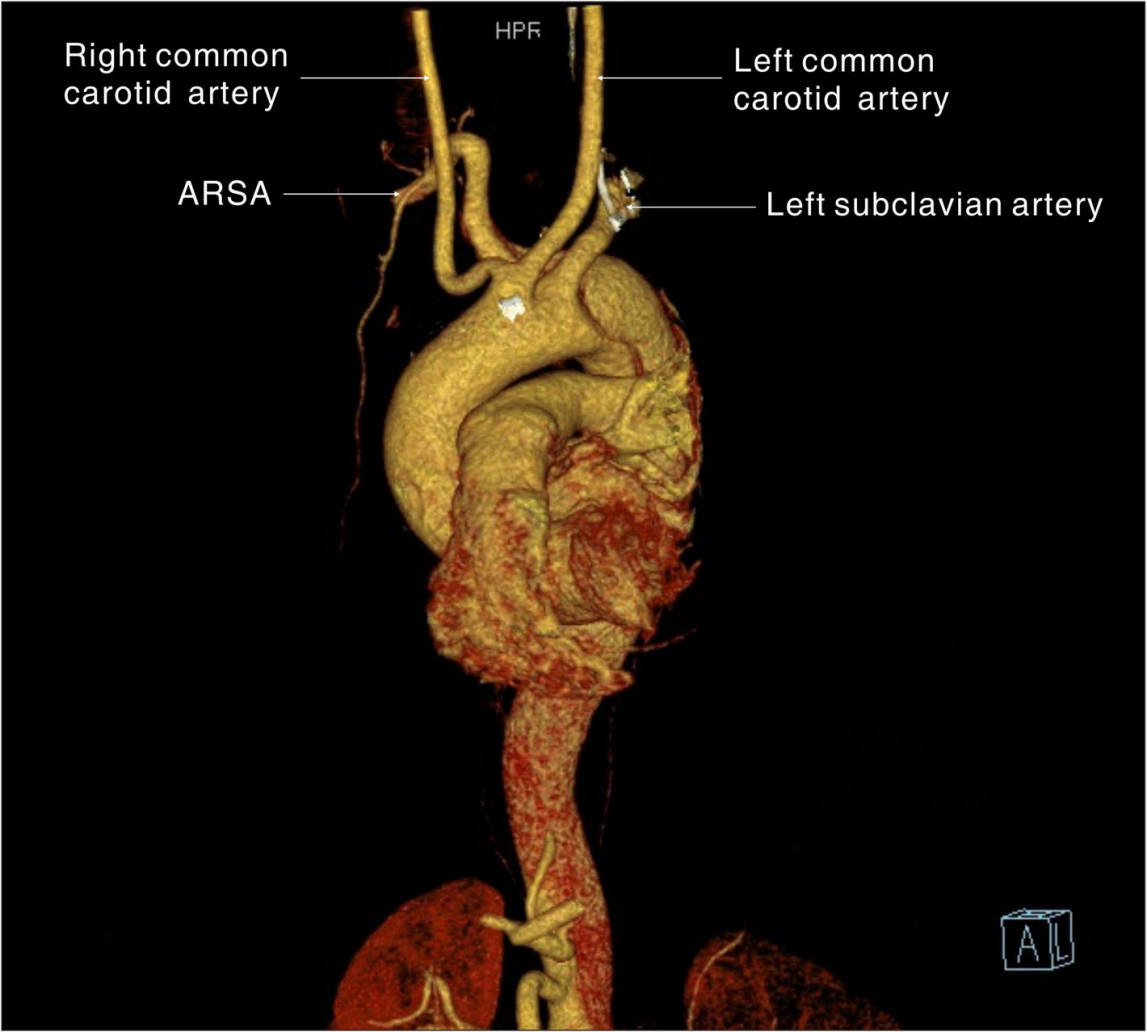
A 3D computed tomography reconstruction shows the aberrant right subclavian artery running distally to the left subclavian artery
